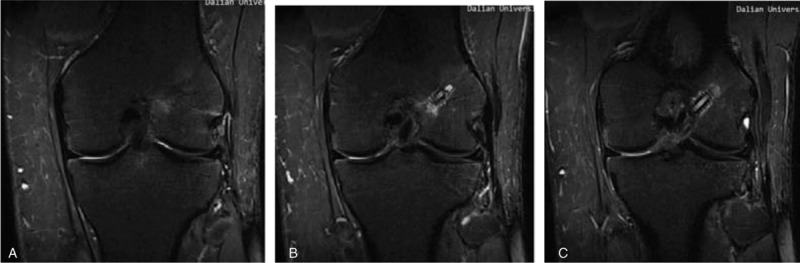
On oblique coronal MRI. MRI = magnetic resonance imaging.
